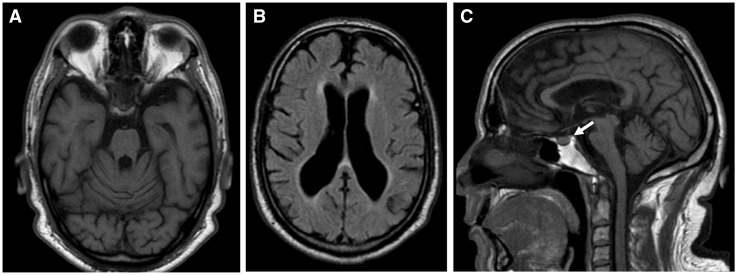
Brain magnetic resonance imaging of the proband. (A) Axial T1. Marked brainstem and cerebellar atrophy. (B) Axial T2 FLAIR. Cortical and subcortical atrophy with ventricular enlargement. (C) Sagittal T1. Brainstem and cerebellar atrophy, and lack of the normal posterior hypophysis hyperintense signal (arrow).
